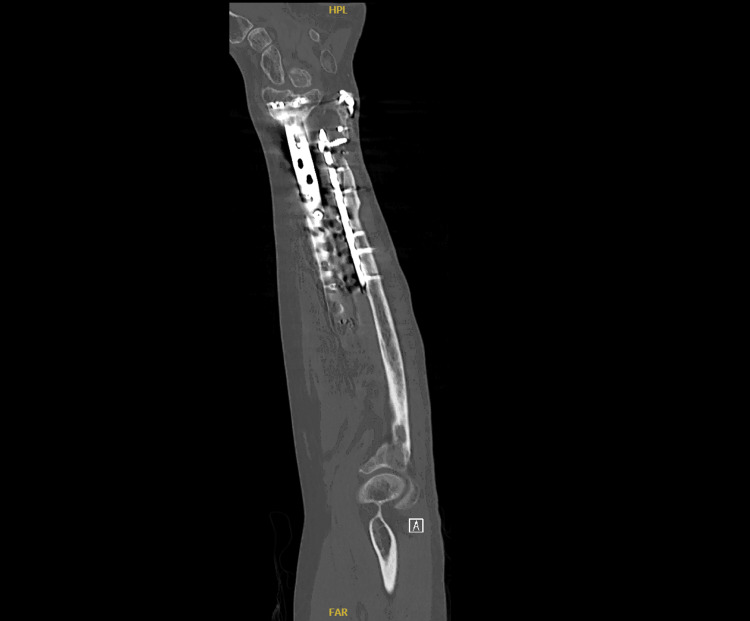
Computed tomography (CT) image of the left forearm showing a healed fracture with a stable implant at the one-year follow-up.
